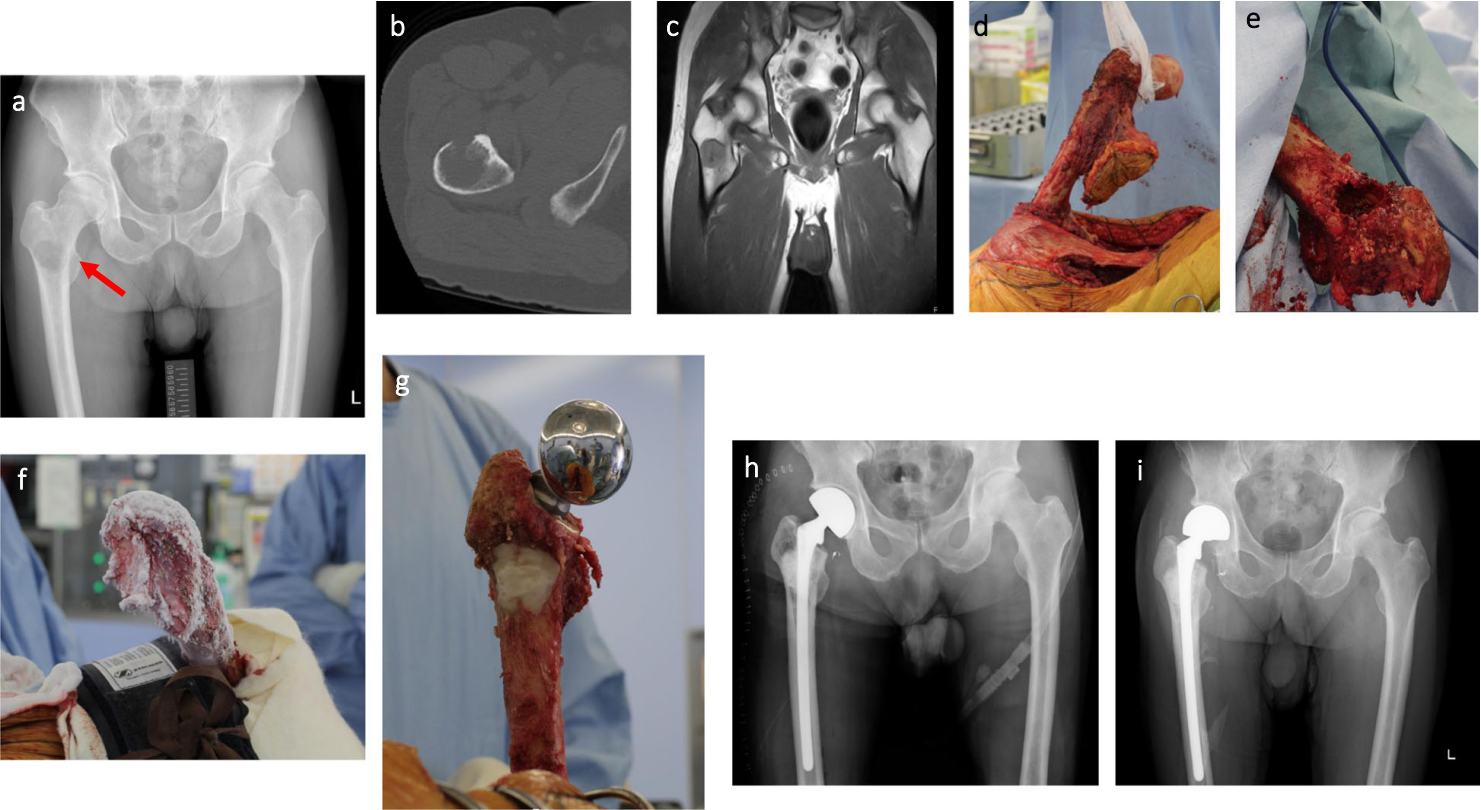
Case 17, a 76-year-old male with RCC (arrow) of right femur treated by pedicle freezing. a. Radiograph of preoperative X-ray. b, c. CT and MRI scan. d. Joint dislocation and exposure of proximal femur after all of soft tissue were detached. e. Resection of biopsy tracts and curettage of bone tumor. f. Thawing at room temperature. g. reconstruction by frozen autograft combination with prosthesis and bone cement. h. Radiograph after immediate operation. i. Radiograph after operation 61 months
